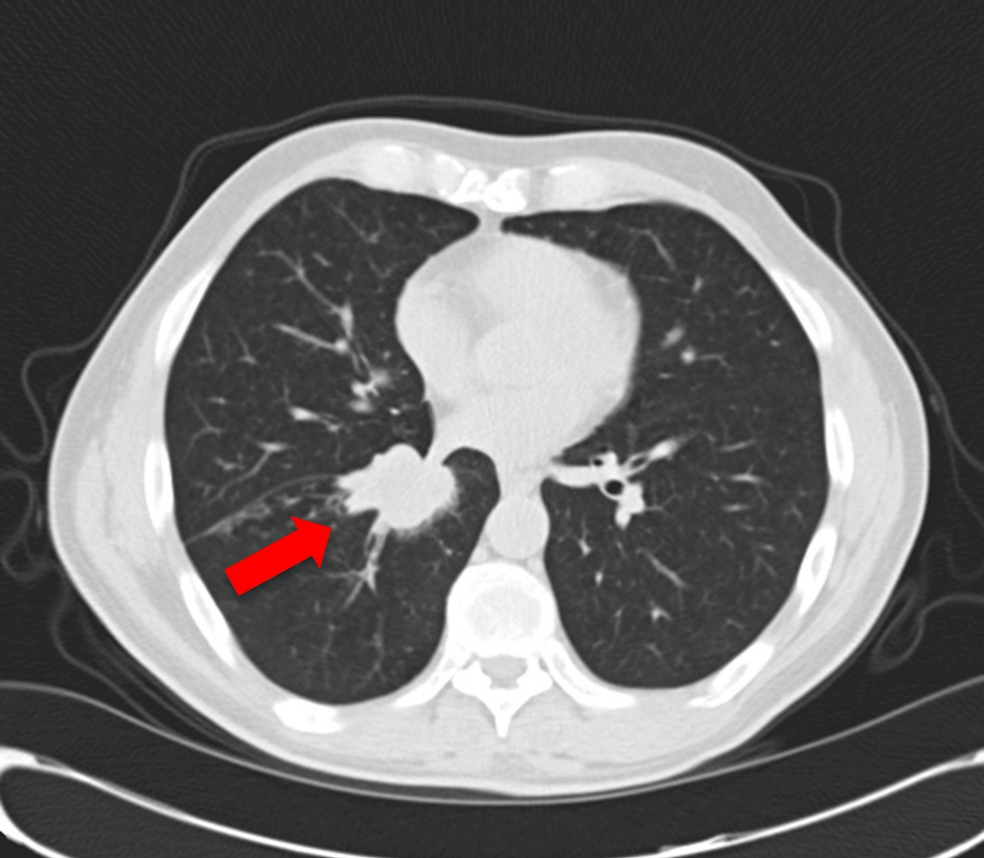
An axial view of the thoracic CT scan reveals a hyperdense mass in the right lung, shown by the arrow.
